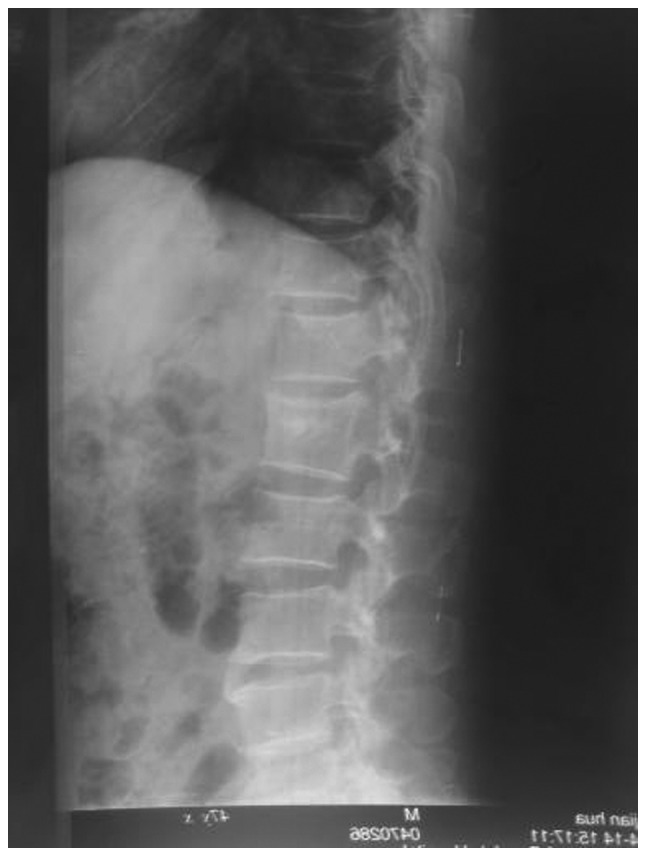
X-ray film following removal of the internal fixation at one year after surgery of a 45-year-old male.
